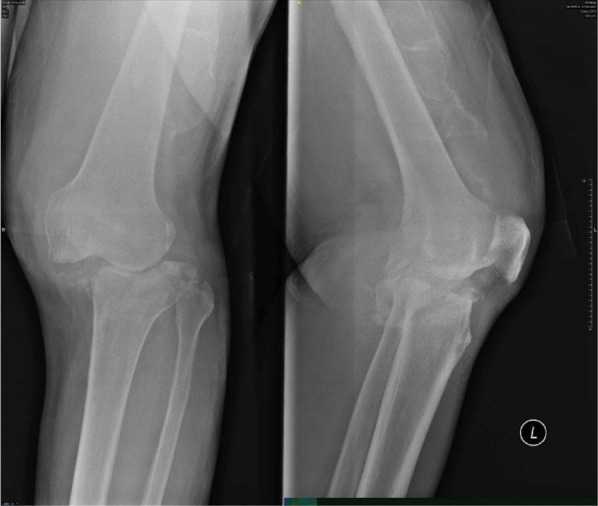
Radiographs AP and lateral views of the left knee, 2 months post-arthroscopic washout and antibiotuc therapy, shows some reformation of the medial femoral and tibial condyles.
